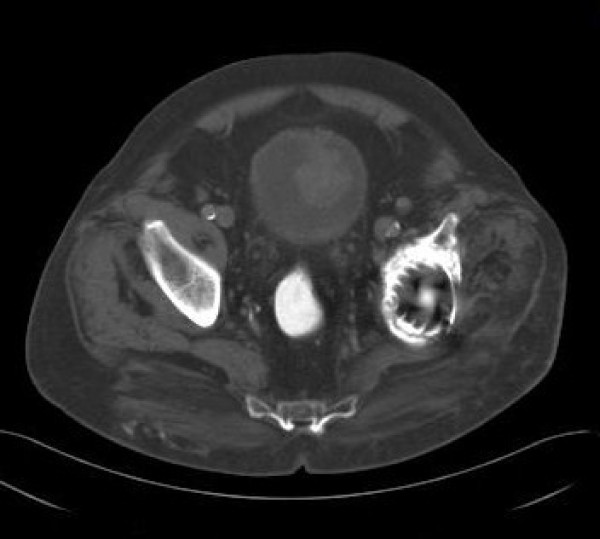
Computed tomography scan shows a large solitary polypoid tumour arising from the bladder dome. Also notice left hip arthroplasty.
